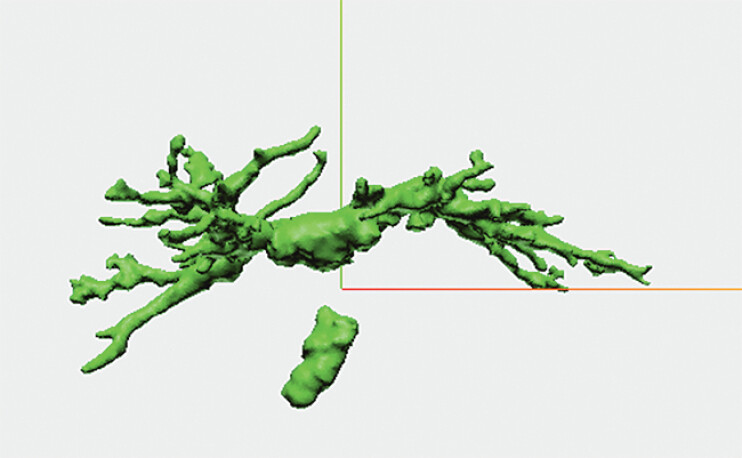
Computed tomographic imaging converted into 3D polygon data.
